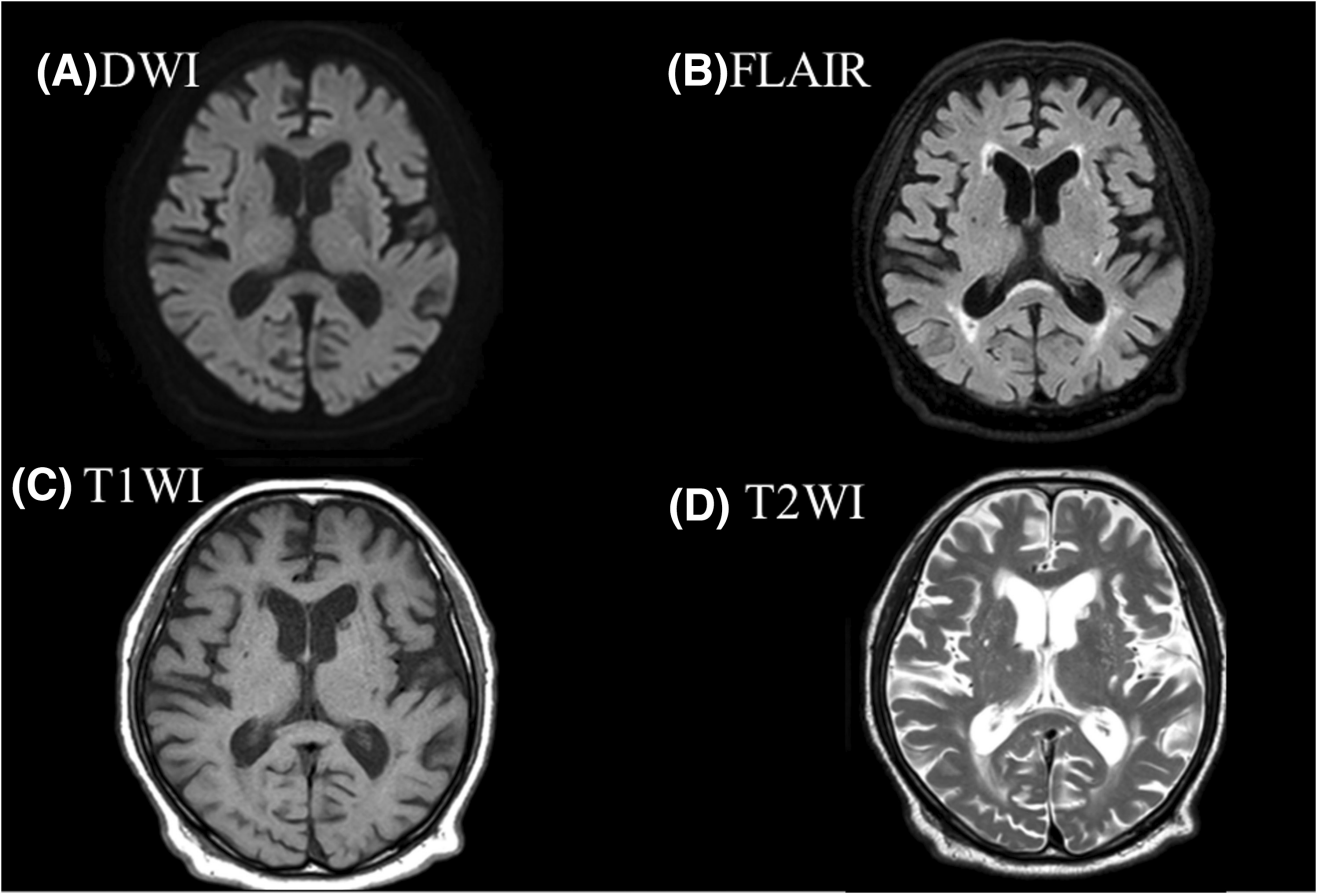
Head magnetic resonance imaging. (a) DWI, diffusion‐weighted imaging; (b) FLAIR, fluid‐attenuated inversion recovery; (c) T1WI, T1‐weighted image; (d) T2WI, T2‐weighted image. There is no image of a stroke.
